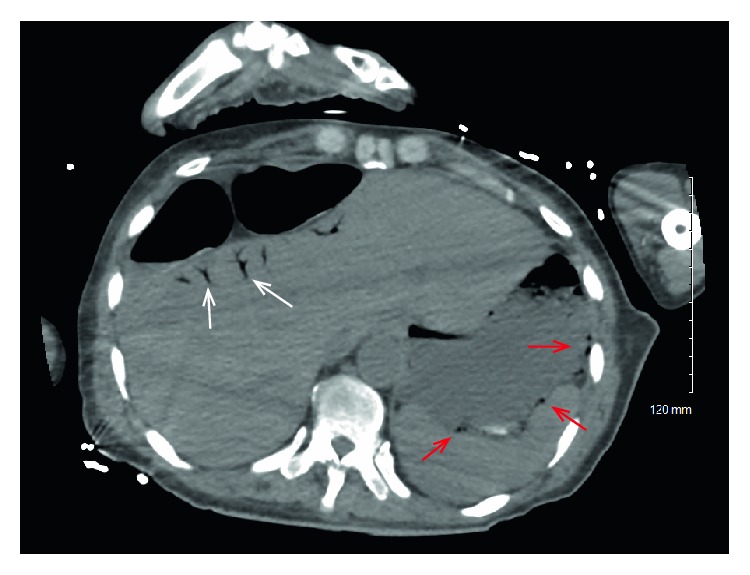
CT findings revealing hepatic portal venous gas (white arrows) and gastric pneumatosis (red arrows).
